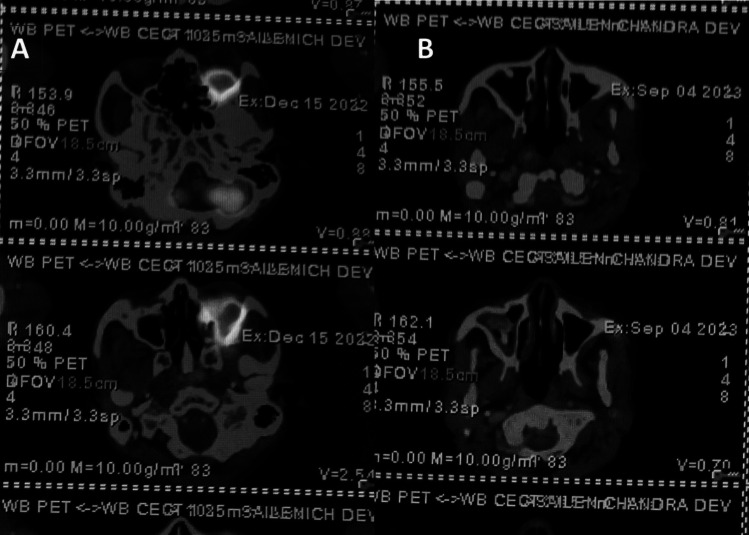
PET-CT images comparing pre-treatment and post-treatment. a Pre-treatment images showing the disease; b 6-month post RT showing complete resolution of the disease
